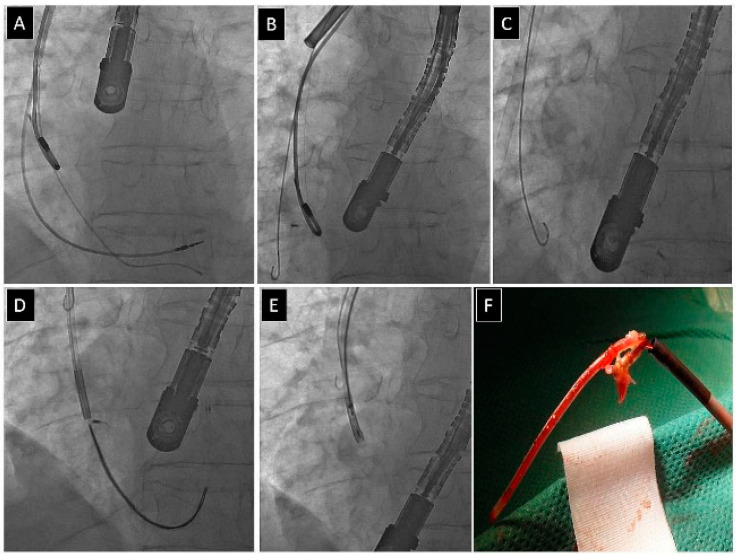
Removal of a DDD pacing system with an abandoned unipolar lead (A). Unipolar lead stretching, little progress despite using a mechanical rotational (TightRail) sheath (B). Bipolar atrial lead was extracted. Attempted extraction using a polypropylene catheter resulted in fracture of the lead, with dissection of its tip (C), which detached from the lead and “danced” to the right ventricle first (C) and then to the right atrium (D). A dozen or so openings and closings of the lasso catheter made it possible to grasp the “tip” of the lead and hide it in the “mother” catheter (Attain CS sheath) (E). X-ray image does not always reflect the size of the remnant. A fragment of the silicone tube made it possible to grasp the “tip” of the lead (F).
